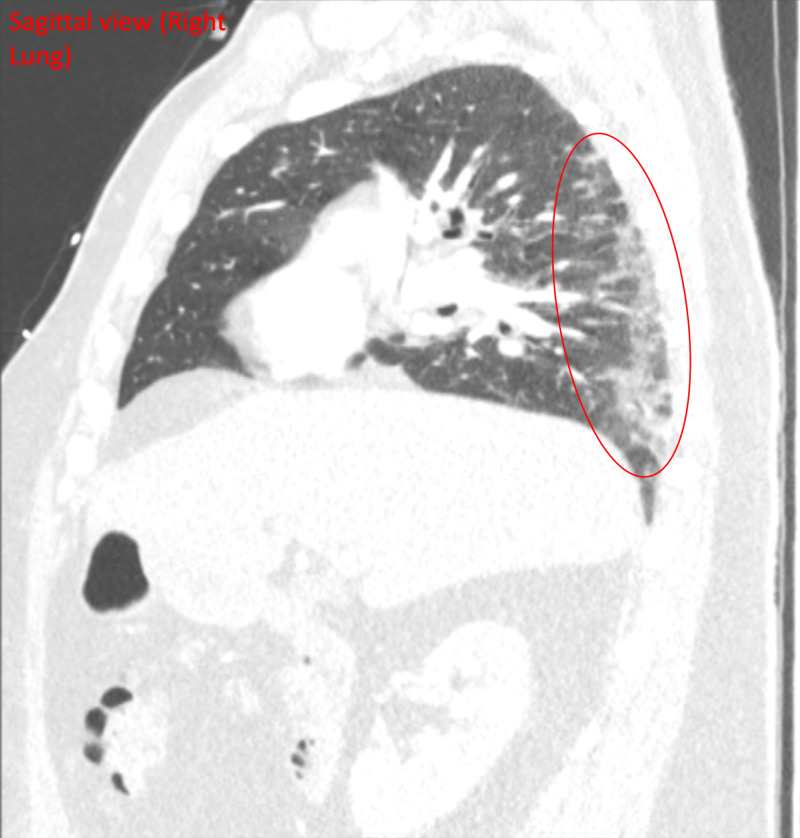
CT chest (sagittal view of the right lung) Multilevel bilateral multifocal peripheral ground-glass opacities (red circle), suspicious for atypical pulmonary infection from bacterial and viral etiology, including COVID-19 CT: computed tomography; COVID-19: coronavirus disease 2019
